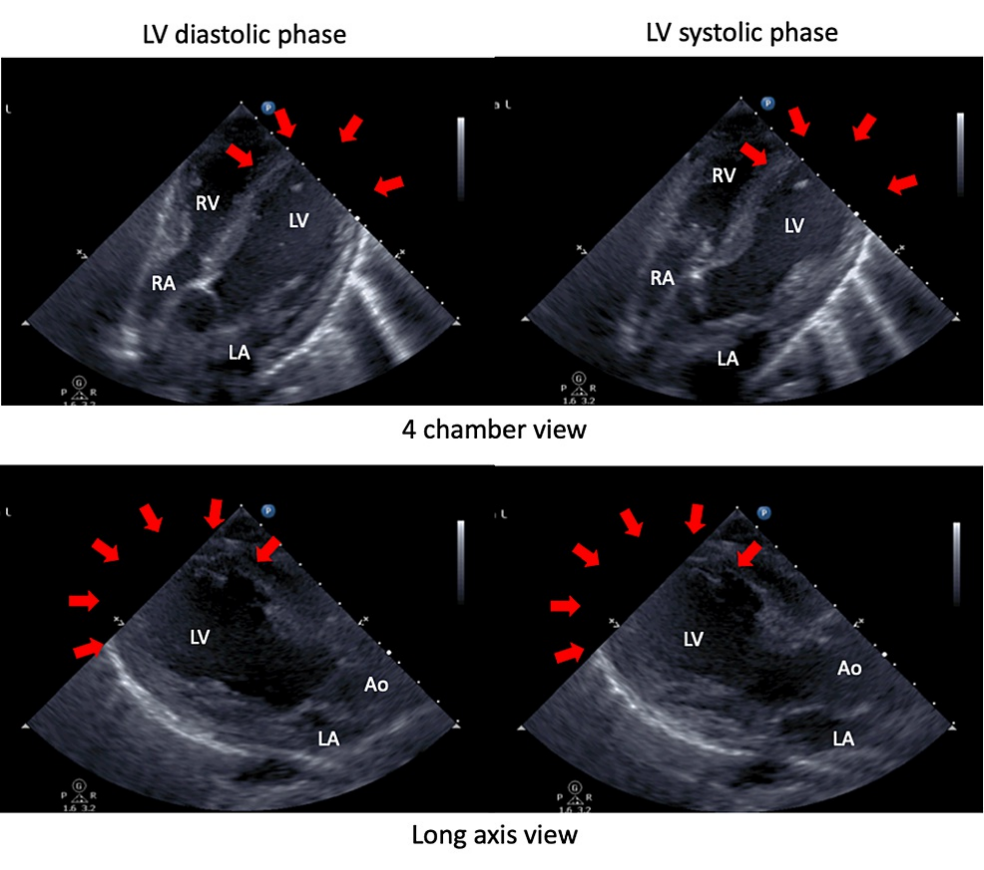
Two-dimensional echocardiogram at admission Echocardiography revealed a severely decreased LV ejection fraction of 20%, with global hypokinesis and apical akinesia (red arrows). End-diastolic (A) and end-systolic (B) frames at admission showed dyskinesia of apex and middle segment of the LV. Ao, aorta; LA, left atrium; LV, left ventricle; RA, right atrium; RV, right ventricle.
